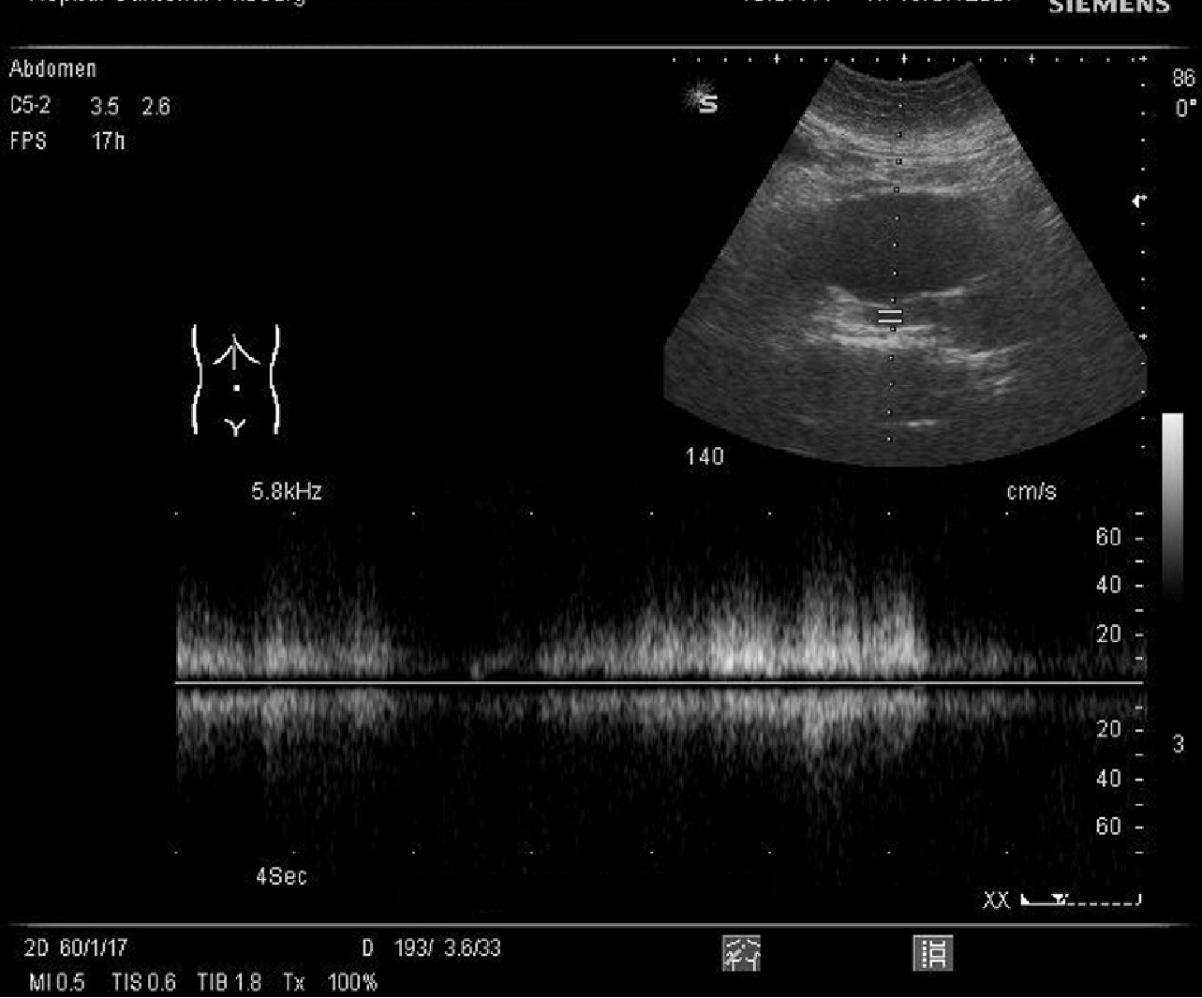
Abdominal ultrasound at the emergency department demonstrating the presence of a vascular structure behind the abdominal aortic aneurysm with a mixed arterio-venous flow due to the arterio-venous fistula.
